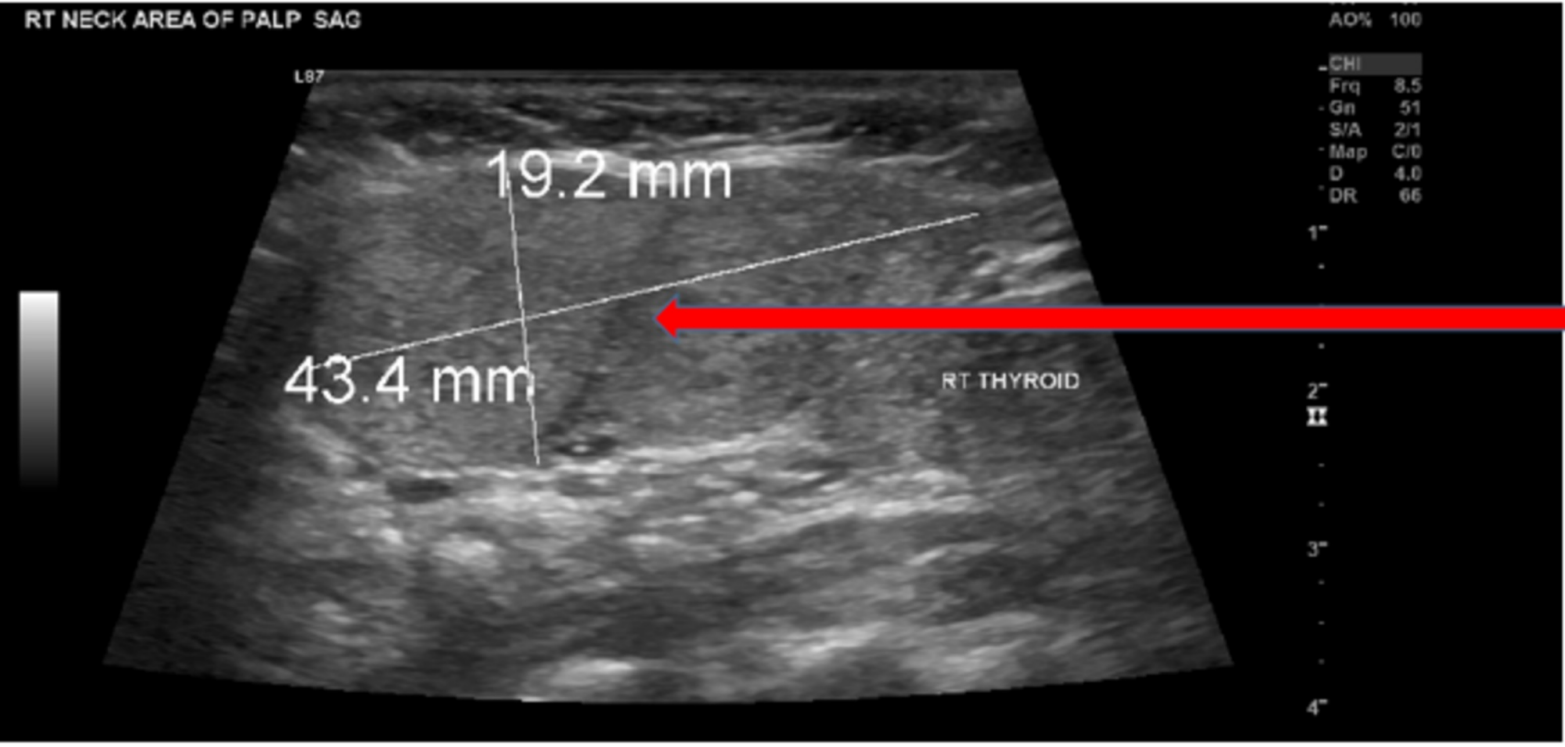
Ultrasound image of the neck Ultrasound image of the neck demonstrating the location of the ectopic thyroid tissue (Red arrow).
